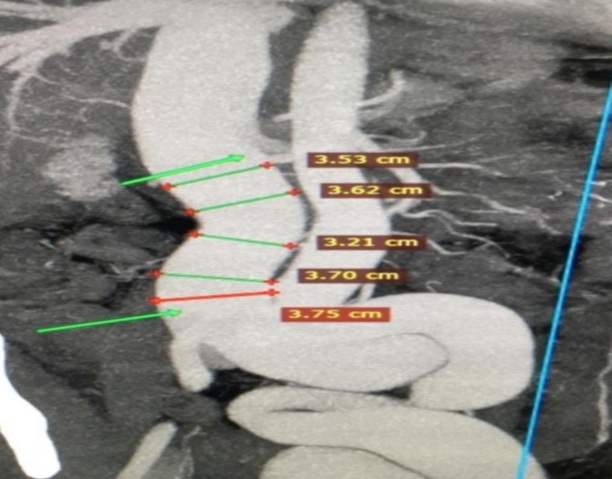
Computed tomography angiography showing dilation of the inferior vena cava measuring up to 37.5 mm in diameter at the iliac vein confluence.
